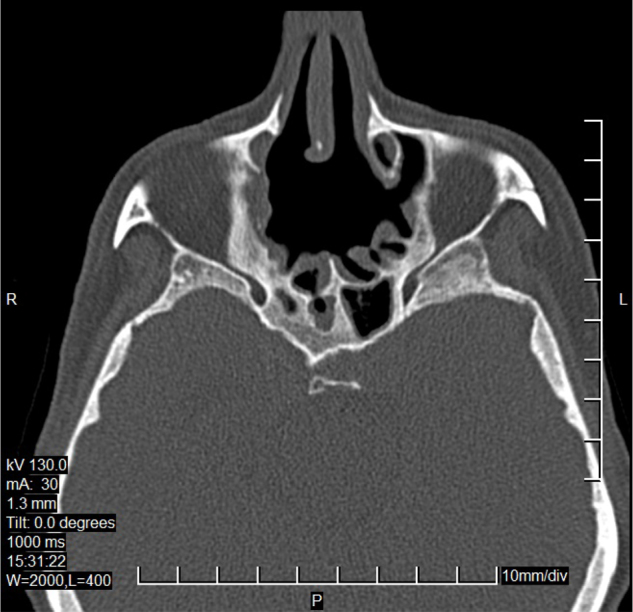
CT scan of the sinuses – 12 months after surgery, axial plane.
